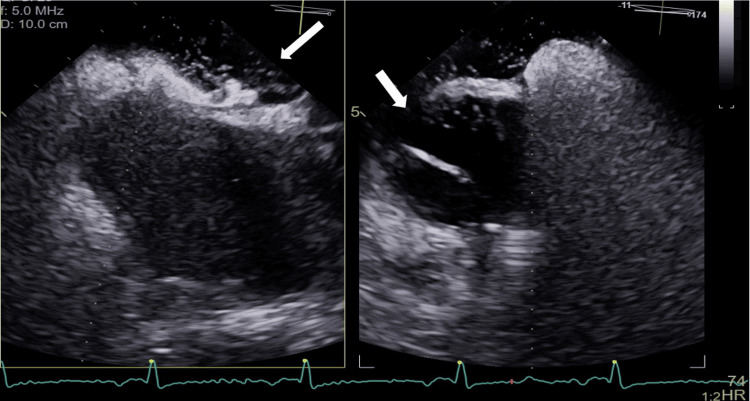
Transesophageal echocardiogram. Interatrial septum with patent foramen ovale (arrow) (A). Right-to-left shunt after administration of agitated saline solution, with passage of more than 30 bubbles in the first 3 cycles (B).
